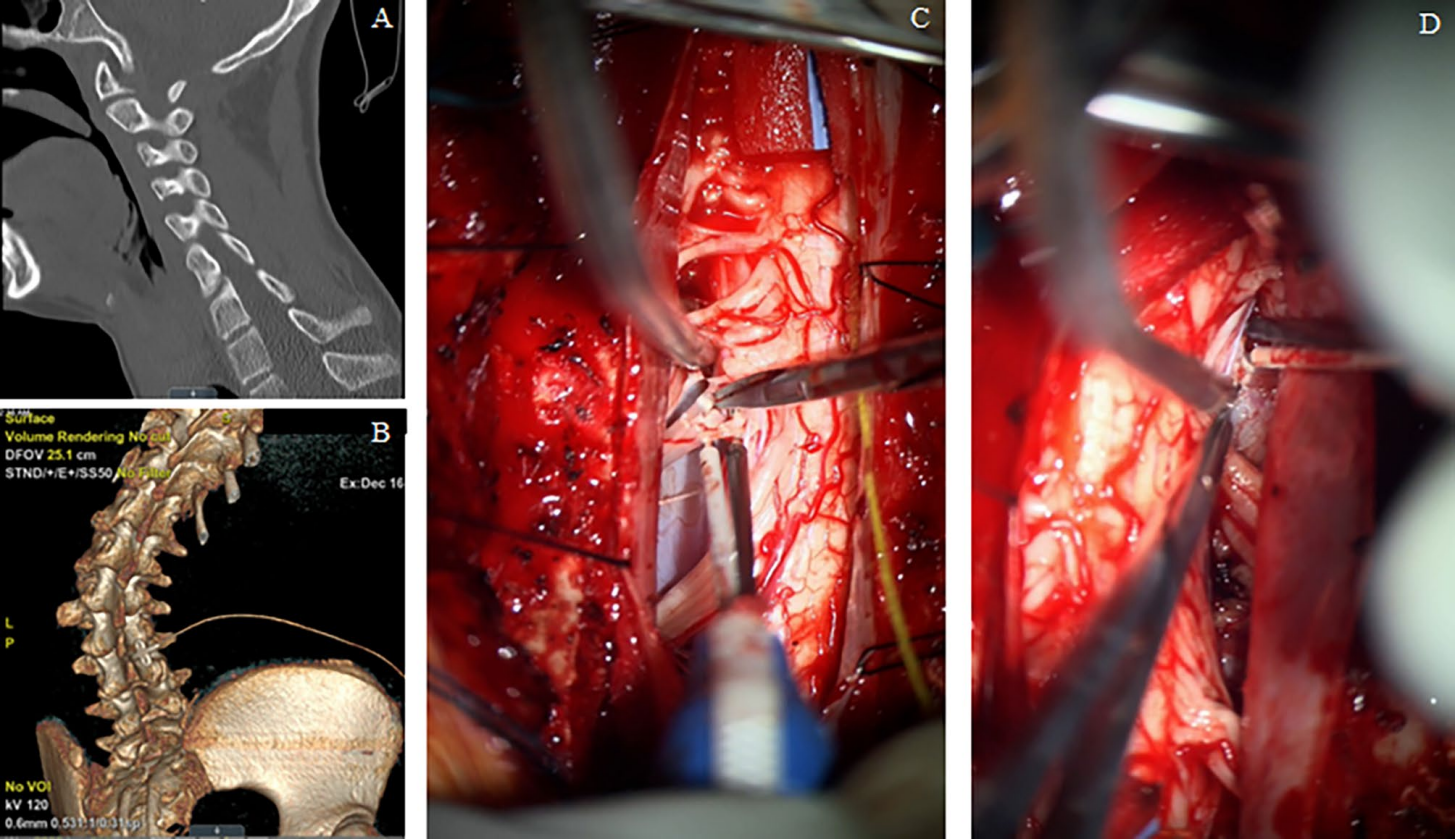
A Lower cervical midsagittal CT demonstrates cervical scoliosis; B 3-D reconstructed lumbosacral CT demonstrates severe rotatory scoliosis and ITB spinal segment C left dorsal and ventral roots easily identified; D spinal cord rotation caused by scoliosis makes the right dorsal and ventral nerve roots both difficult to access
